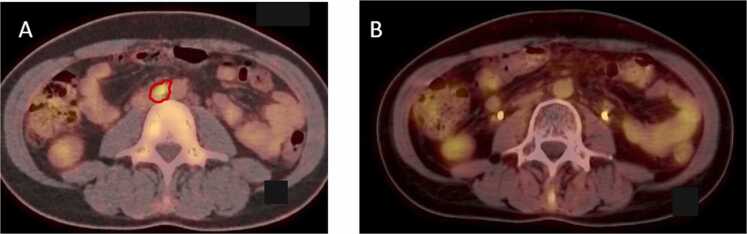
18F-FDG PET/CT images showing metabolic response of para-aortic lymphadenopathy. (A) Pathological hypermetabolic uptake (outlined in red) involving the para-aortic lymphadenopathy, which was progressing after conventional chemoradiotherapy and was not included in the carbon ion boost treatment plan. (B) A complete metabolic response at this site on the first PET scan performed 6 months after the boost.
